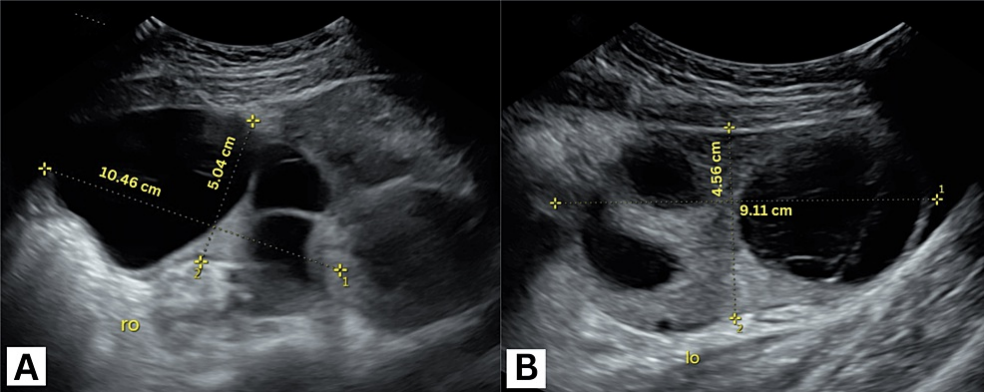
Transabdominal ultrasound images showing bilateral enlarged ovaries with multiple cysts; (A) right ovary (ro) and (B) left ovary (lo).
